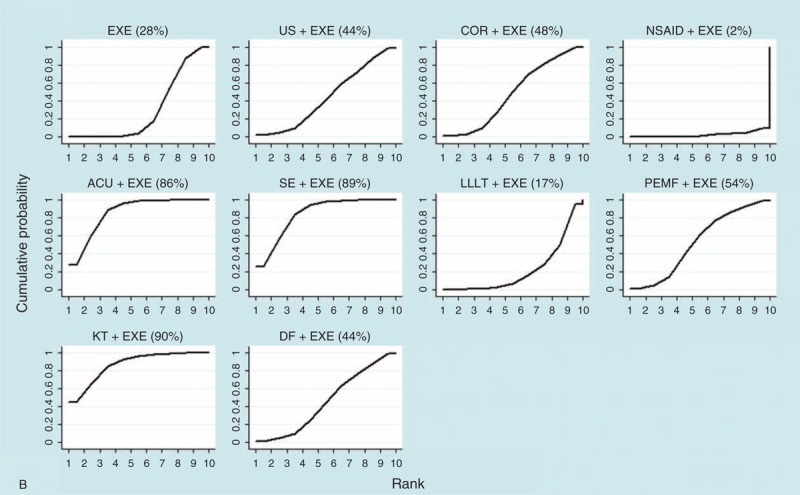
(A) SUCRA for nonoperative treatments (pain score). (B) SUCRA for nonoperative treatments (CMS). (C) SUCRA for operative treatments (pain score). (D) SUCRA for operative treatments (CMS). Note: The area under the curve represents the cumulative rank probability of each treatment, with larger areas signifying higher probabilities. ACU = acupuncture therapy, ASD = routine arthroscopic subacromial decompression, COR = corticosteroid injection, DF = diacutaneous fibrolysis therapy, EXE = routine exercise treatment, HYA = hyaluronate injection, KT = kinesio taping therapy, LLLT = low-level laser therapy, MAN = manual therapy, MWD = microwave diathermy therapy, NON = no treatment/placebo, NSAID = nonsteroidal anti-inflammatory drug injection, onlyBUR = arthroscopic bursectomy without acromioplasty, OSD = open subacromial decompression, PEMF = pulsed electromagnetic field therapy, PLG = platelet-leukocyte gel injection, rESWT = radial extracorporeal shockwave therapy, RF = radiofrequency therapy, SE = specific exercise therapy, US = ultrasound therapy.
